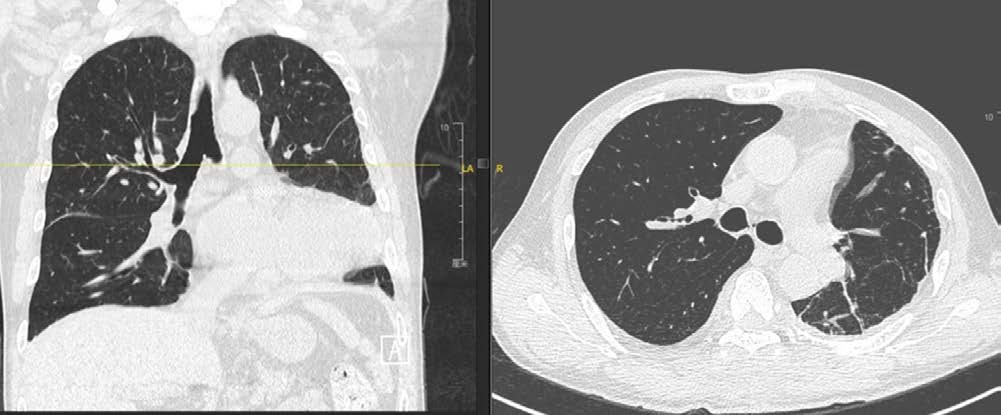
Postoperative chestcomputed tomography (CT) on day 10. CT = computed tomography.
